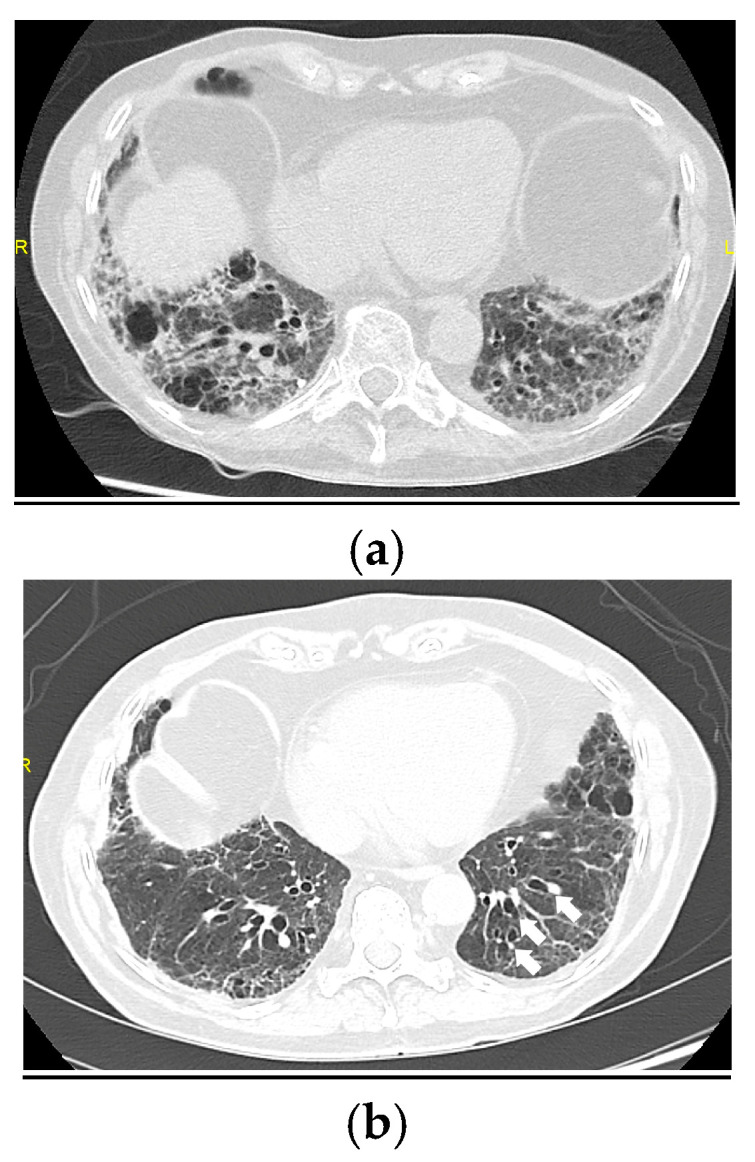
(a) Follow-up HRCT at 6 months after hospital discharge. Image showing ground-glass opacities and septal thickening in both lower lobes, with cylindrical bronchiectasis in the right lower lobe. (b) Follow-up image at 12 months showing a radiological improvement of the ground-glass opacities, but with the development or worsening of bronchiectasis in the left lower lobe (arrows); the findings are suggestive of post-COVID-19 sequelae.
